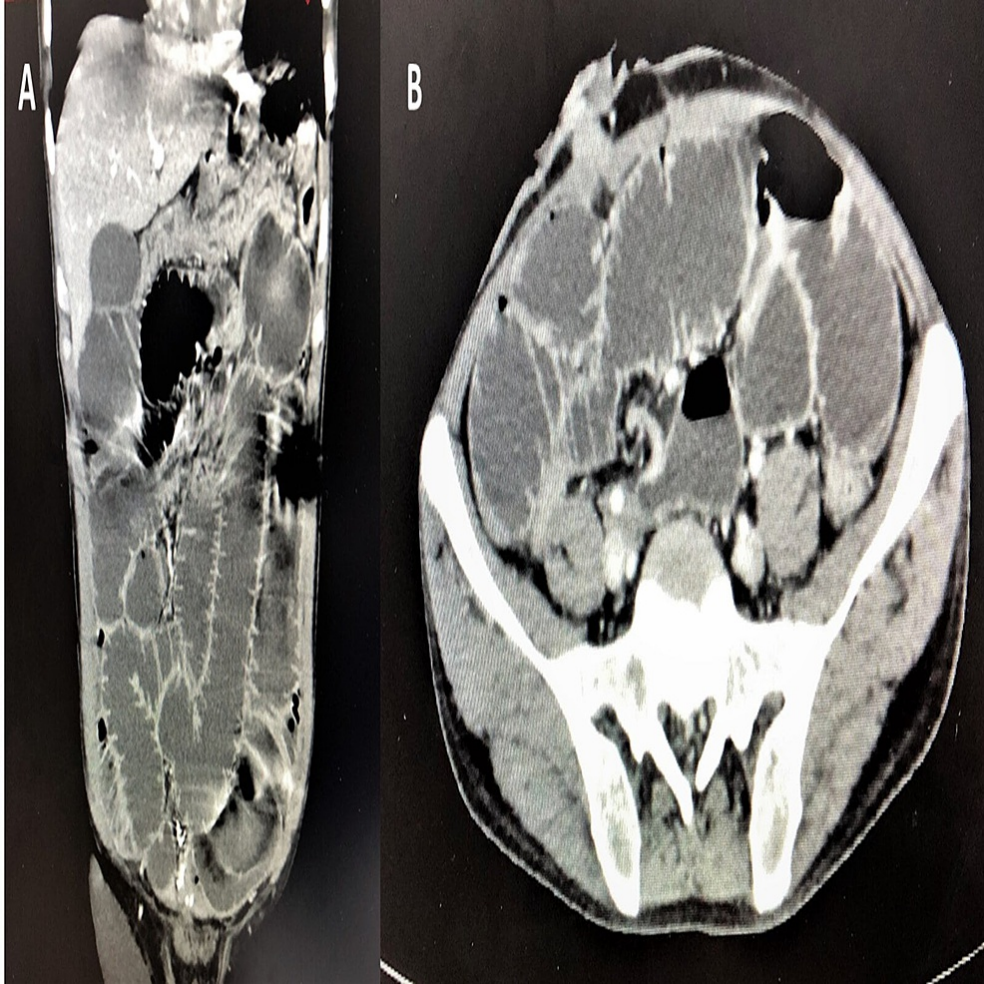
CECT of the abdomen coronal (A) and axial (B) views revealing dilated small bowel loops of jejunum and proximal ileum with transition point in the distal ileum, with collapsed large bowel. CECT: contrast-enhanced computed tomography
